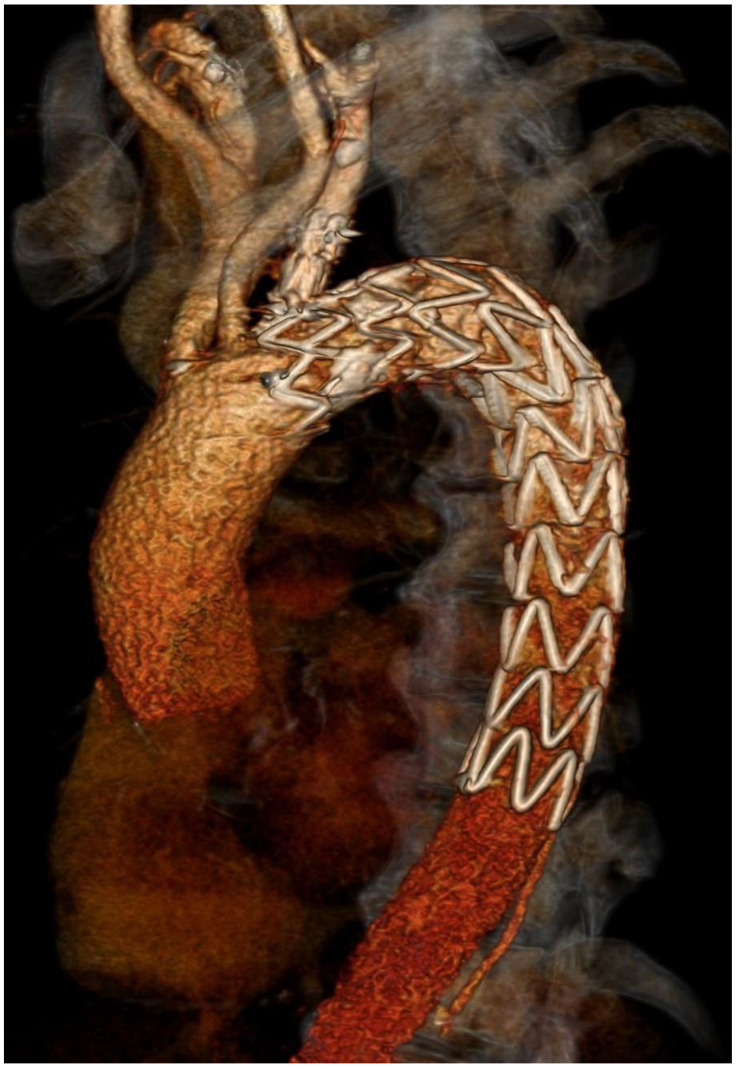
Postprocedural 3D CT showing Castor stent graft.
